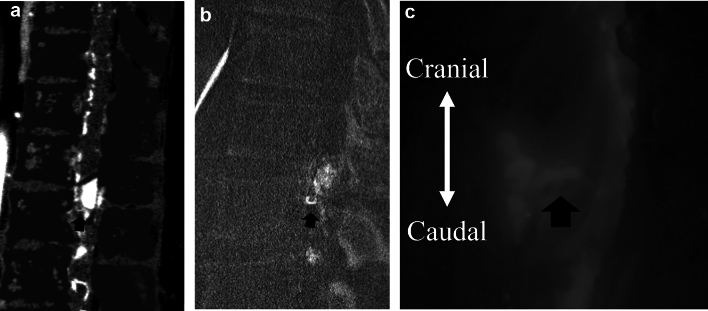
Spinal hemangioblastoma. A case of a male patient in his 60 s with lower back pain, gait disturbances, and pain in both the lower limbs. MRI T2WI showed an iso-intensity mass and flow voids around the mass. The PCD-CTA shows several masses (max 18 mm) with strong contrast effects with dilated blood vessels flowing into or out of the mass and a tiny curve vessel extending anteriorly from the mass in the sagittal view (a). The slab MIP image sagittal view of 3D angiography (b) shows a feeder from the anterior spinal artery and a draining vessel from the masses, which was almost equal to PCD-CTA. Intraoperative findings show a feeder from the anterior spinal artery, in contrast to the shiny structure in the ICG (c). *MRI* magnetic resonance imaging, *T2WI* T2-weighted imaging, *PCD-CTA* photon-counting detector-computed tomography angiography, *ICG* indocyanine green
